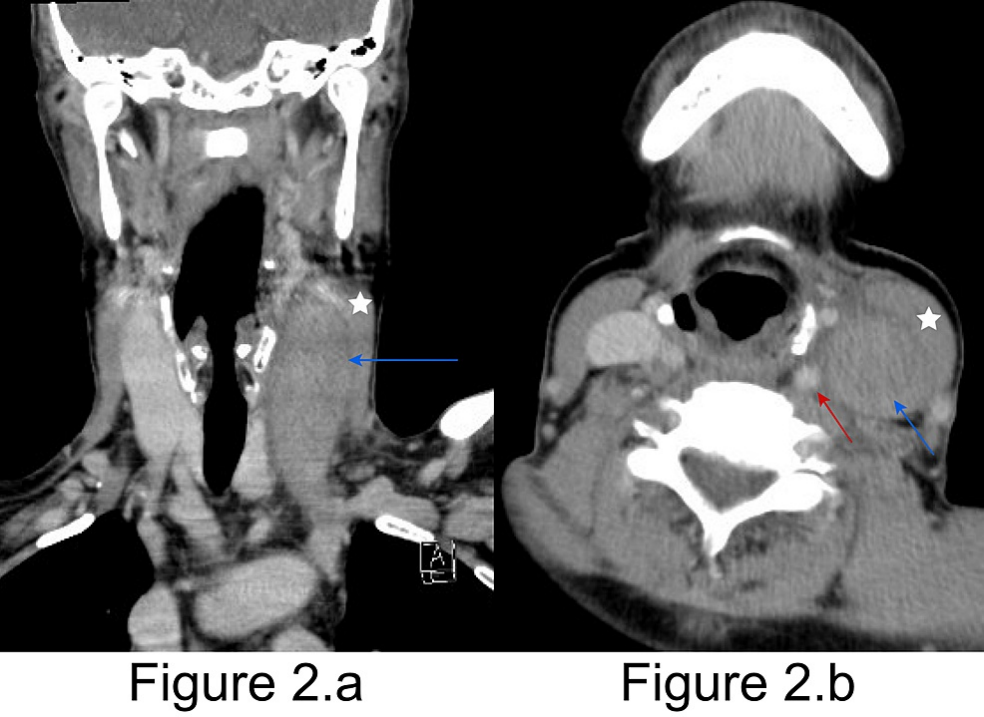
CT neck with contrast 2.a: Coronal view of the CT neck with contrast shows the sternocleidomastoid muscle (star) and the thrombotic left internal jugular vein (blue arrow). 2.b: Axial view of the CT neck with contrast shows the sternocleidomastoid muscle (star), the thrombotic left internal jugular vein (blue arrow), and the left common carotid artery (red arrow).
